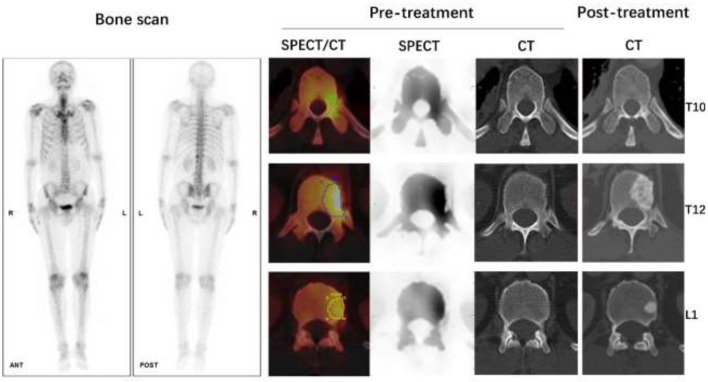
Male, 62 years old, CT guided biopsy of a tumor in the lower lobe of the right lung revealed a moderately differentiated adenocarcinoma, with an EGFR 19 mutation. Before treatment, a full-body bone scan revealed suspicious metastasis of the T10, T12, and L1 vertebrae. The SUVmax of lesions on T10, T12, and L1 vertebrae were 9.71, 14.80, and 10.52 g/ml, respectively, according to quantitative SPECT/CT. But the CT scan of these vertebrae on pre-treatment images showed no abnormal bone density changes. After 4 months of Almonertinib therapy, a post-treatment CT scan revealed increased bone mineral density in the high uptake area of SPECT images, confirmed bone metastasis of these vertebrae.
